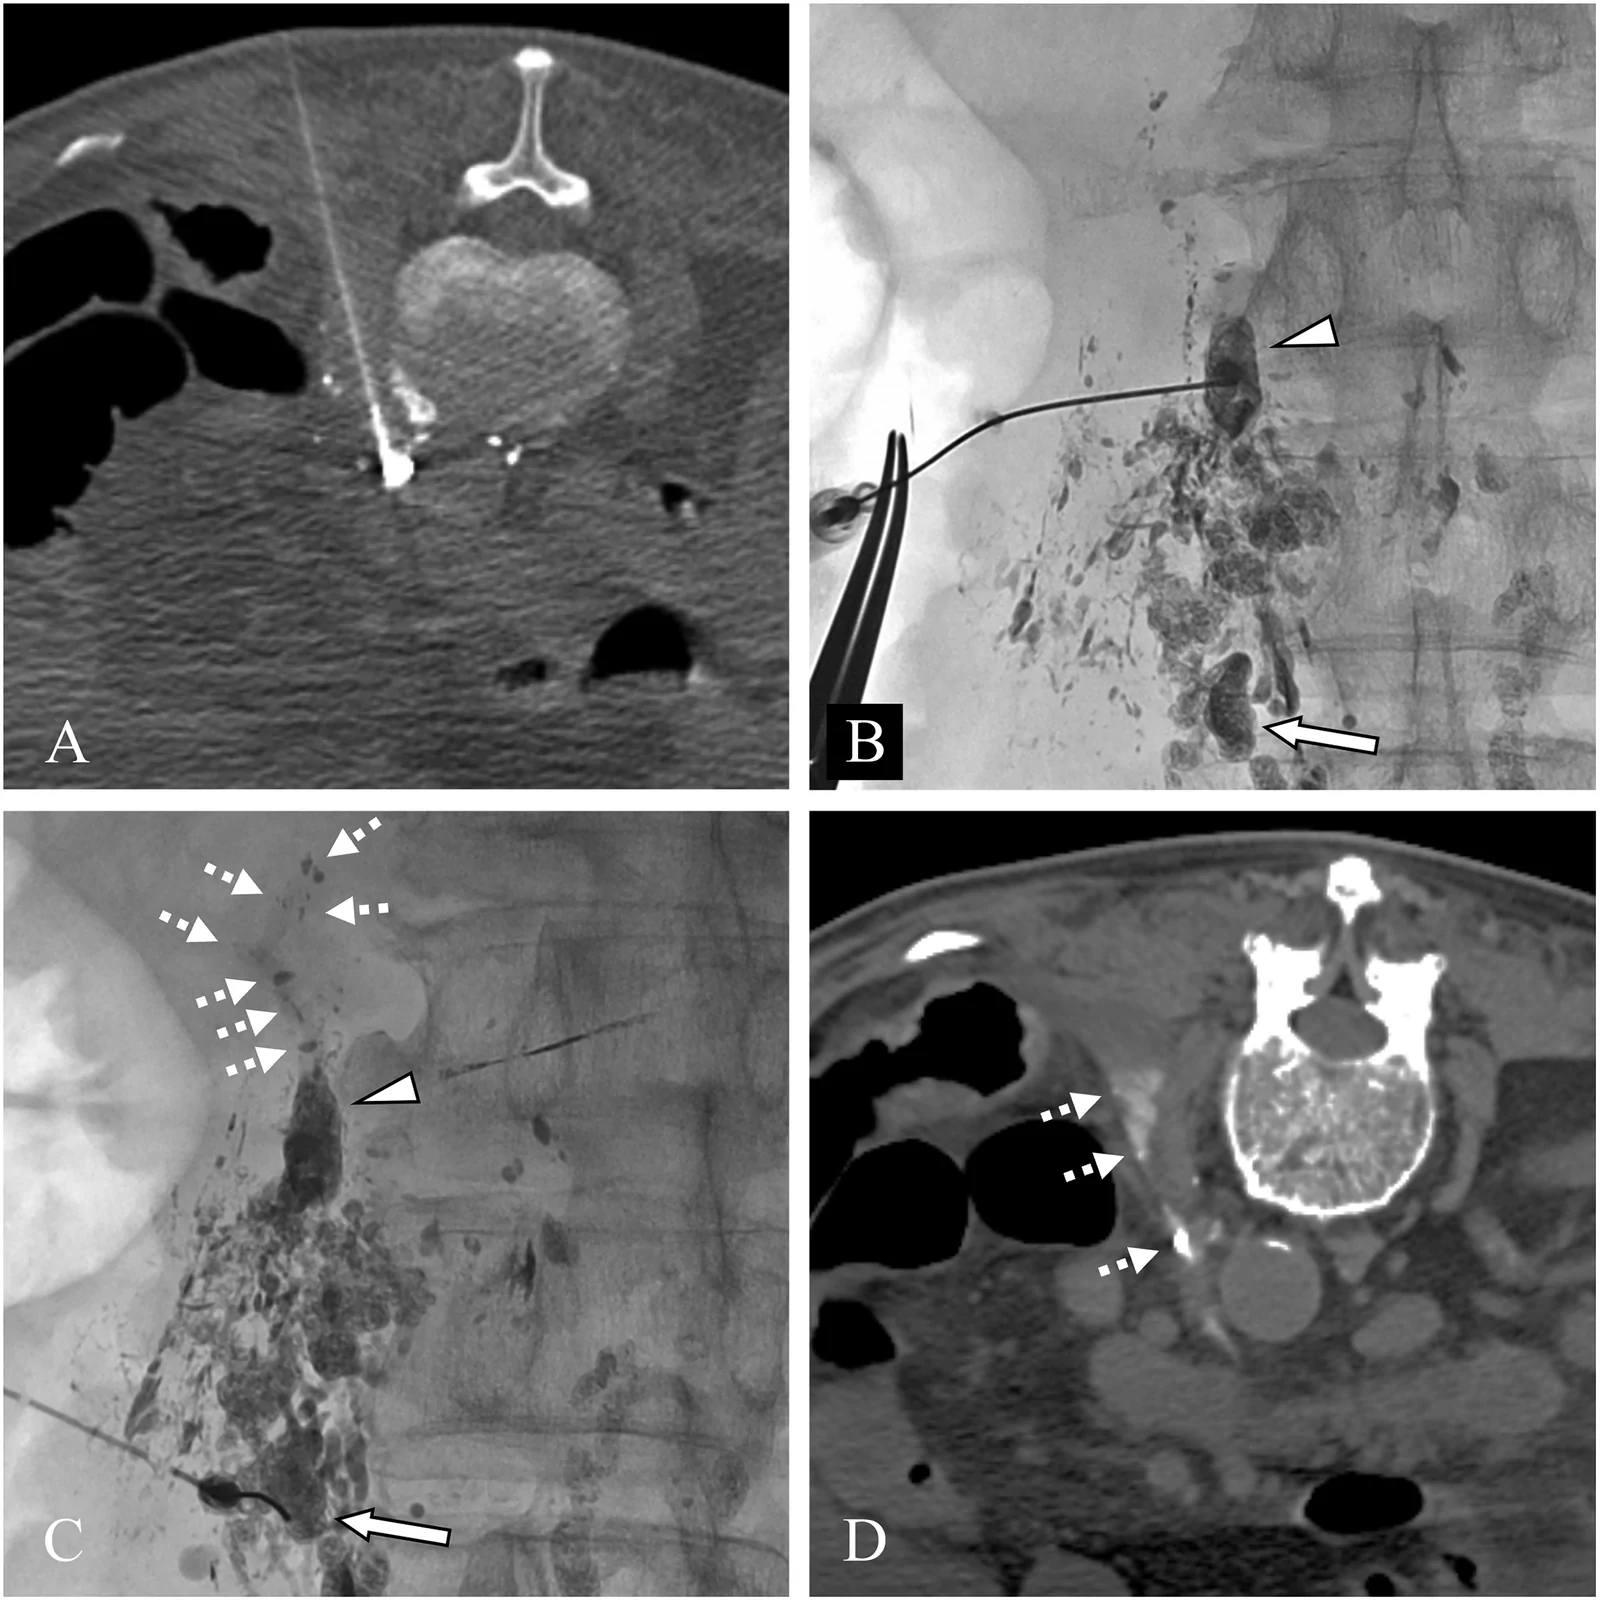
Percutaneous lymphatic embolization. (A) CT-guided puncture of the lymphopseudoaneurysm. (B) Fluoroscopy-guided injection of an NBCA/ethiodized oil mixture for embolization. Notably, the lymphopseudoaneurysm is filled with the NBCA/ethiodized oil mixture (arrowhead). The arrow indicates the caudal para-aortic lymph node. Note that the left and right sides are reversed because the image was acquired in the prone position. (C) CT-guided embolization of the caudal para-aortic lymph node (arrow) using the NBCA/ethiodized oil mixture. Fluoroscopy shows ethiodized oil distribution in the target lymph node, lymphatic pathway, and retroperitoneal space (dashed arrows), appearing as faint hyperattenuating areas consistent with extravasated iodinated contrast. The arrowhead indicates the embolized lymphopseudoaneurysm. Note that the left and right sides are reversed because the image was acquired in the prone position. (D) Post-injection CT shows distribution of ethiodized oil corresponding to the leakage site (arrows).
